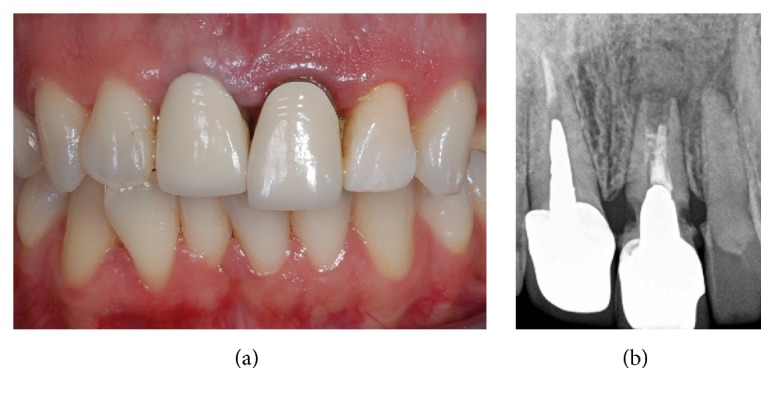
Preoperative situation. The patient complains because the left central incisor, which was restored with a single crown several years before, appears extruded and presents a high mobility (a). The periapical radiograph shows a severe resorption (b): the tooth is nonrestorable and needs to be extracted.
